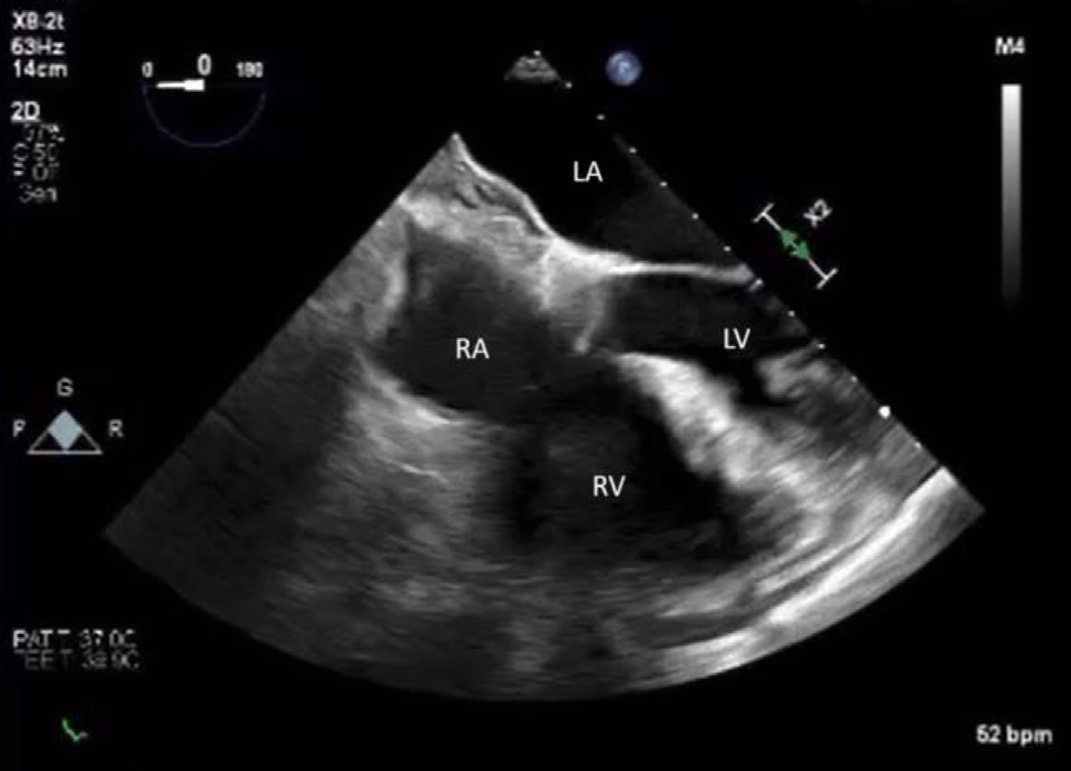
Transesophageal Echocardiography Showing a Thickened Intra-Atrial Septum With Minimal Residual Tumor in the Right Atrium After the Second Aspiration Procedure LA = left atrium; LV = left ventricle; RA = right atrium; RV = right ventricle.
